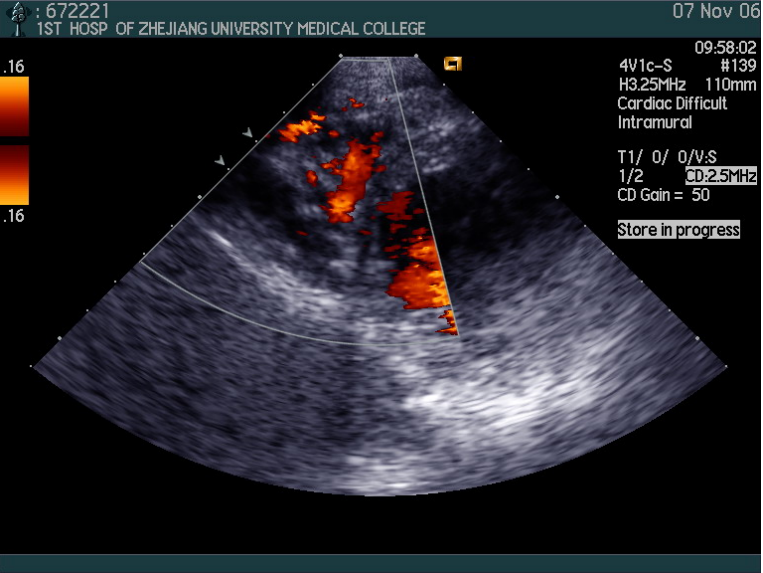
the same view as figure 2 showing the color flow of deep intertrabecular recesses to right ventricular chamber by color Doppler echocardiograms.
